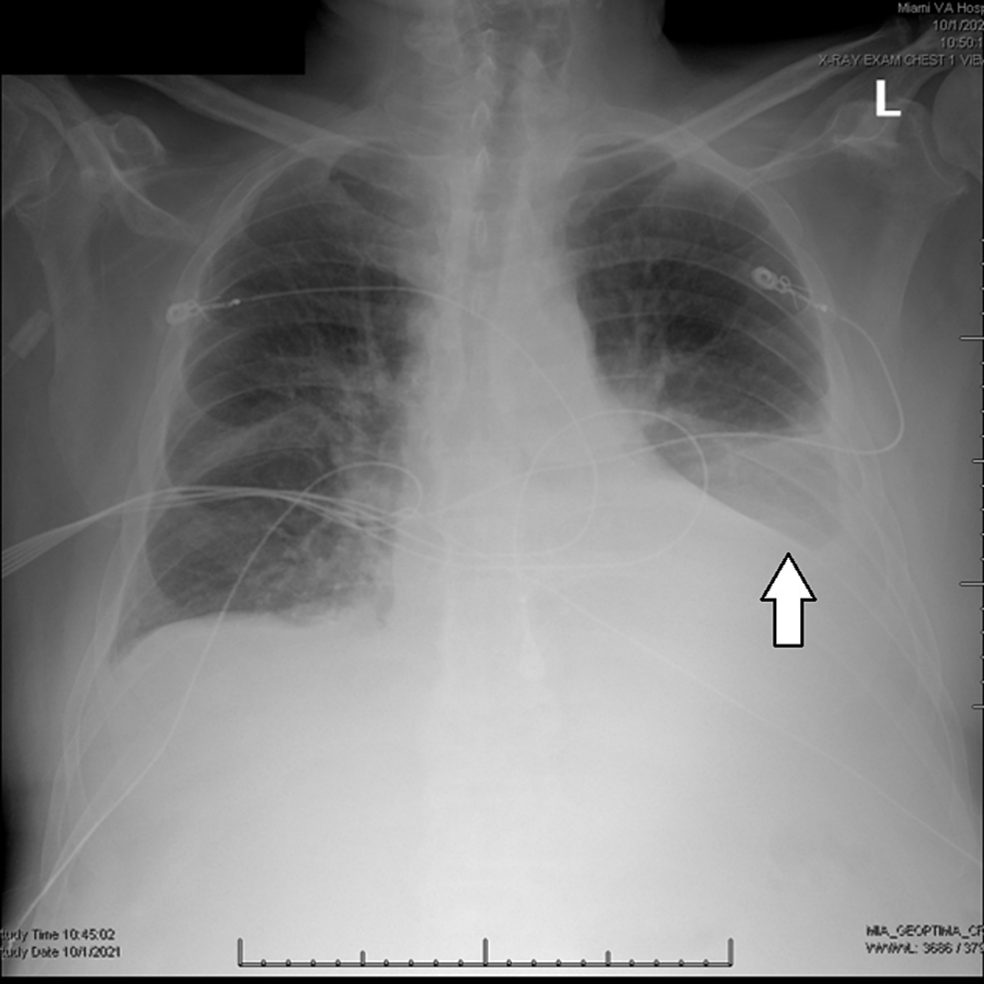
Posteroanterior chest radiograph on admission showing congestive changes, left-sided pleural effusion (arrow), and basilar underaeration.
